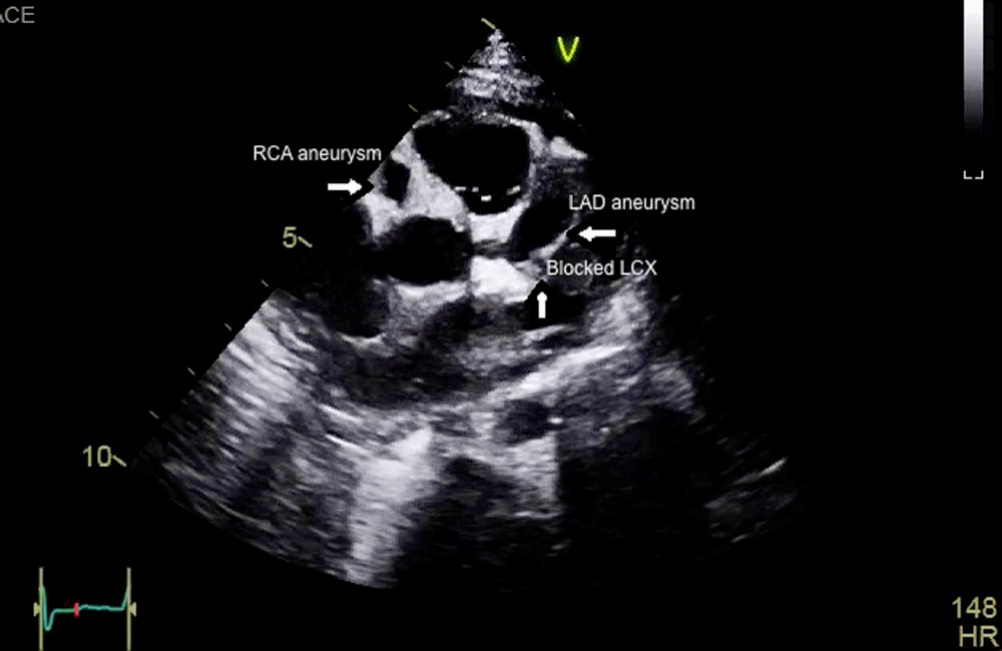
Echocardiography, short-axis view, showing aneurysms in the left anterior descending and right coronary artery, with totally thrombosed left circumflex. Arrows indicate the aneurysmal segments and the occlusive thrombus in the left circumflex artery.
